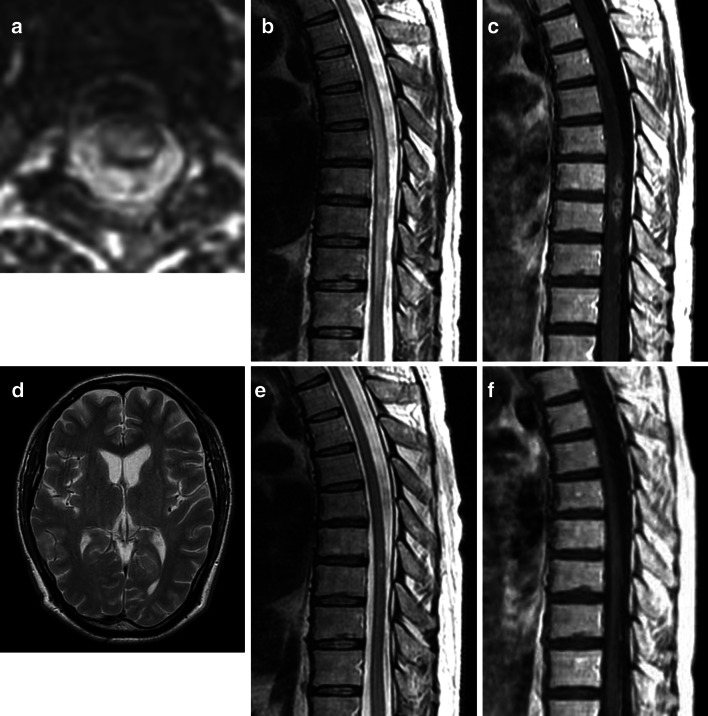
Spine magnetic resonance imaging (MRI) before (a–c) and after (e, f) infliximab therapy and cranial MRI (d). a Axial T2-weighted image showing transverse myelitis. b Sagittal T2-weighted image showing abnormally increased longitudinal T2 signal with mild enlargement from T6 to T10. c Sagittal contrast-enhanced T1-weighted image revealing partial enhancement of the lesion. d Cranial MRI had no abnormal finding. e Sagittal T2-weighted image showing reduced longitudinal abnormal signal. f Sagittal contrast-enhanced T1-weighted image revealing no enhancement lesion
